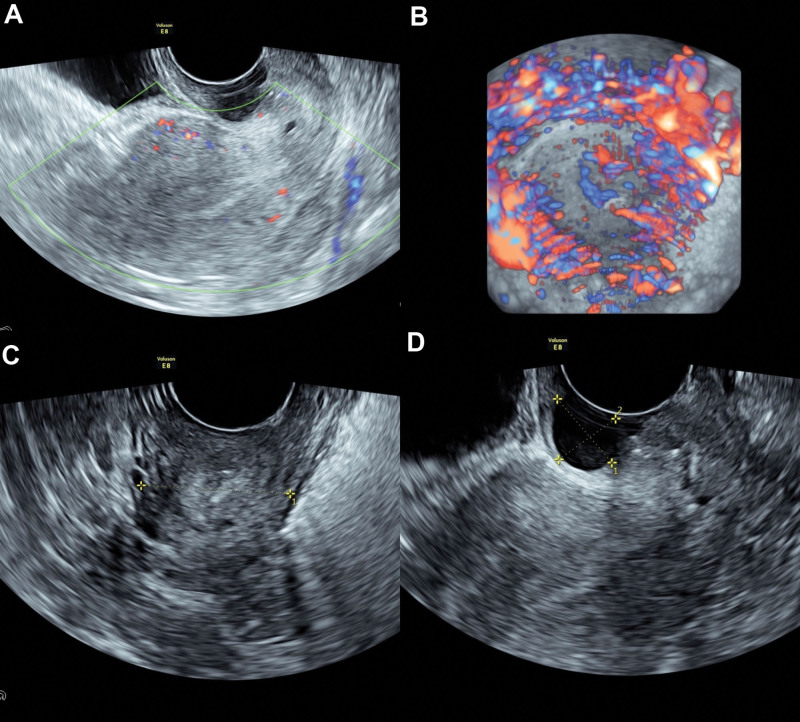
The ultrasound examination suggested a 5.1*4.0*4.4 cm uterus with normal blood supply and endometrium with thickness of 0.7 cm (A); a cervix with uniform blood supply (B) and without space-occupying lesion (C) expect for multiple cervical cysts, whose maximum size was 1.6*1.3 cm (D).
